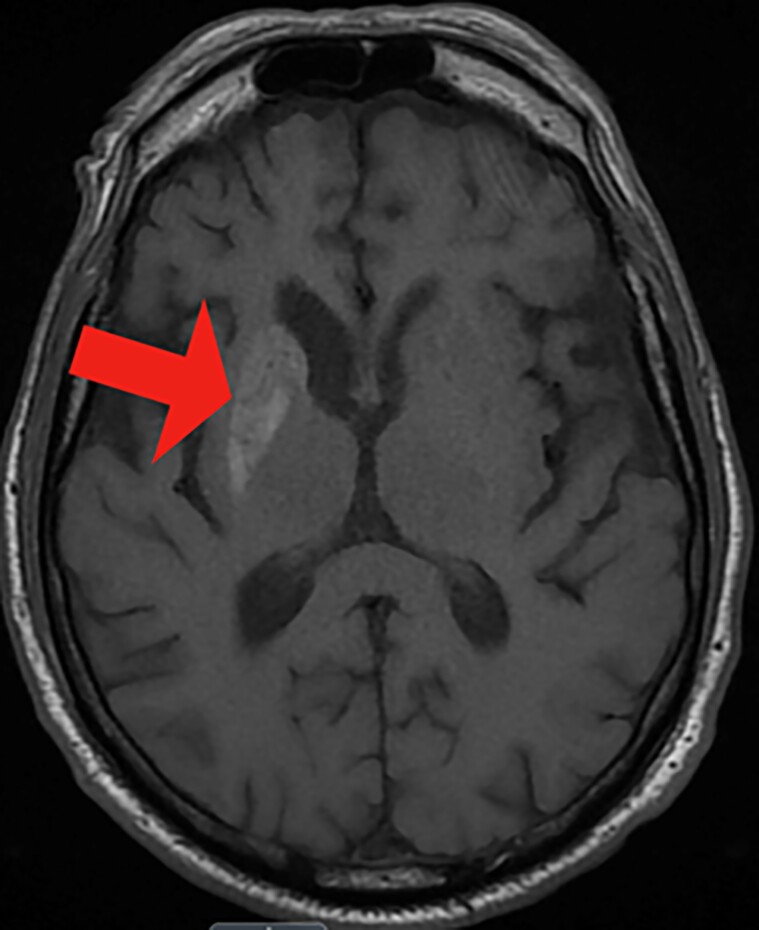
MRI brain: T1 shortening involving the right caudate and putamen corresponding to the hypodensity seen on CT scan (red arrow).
